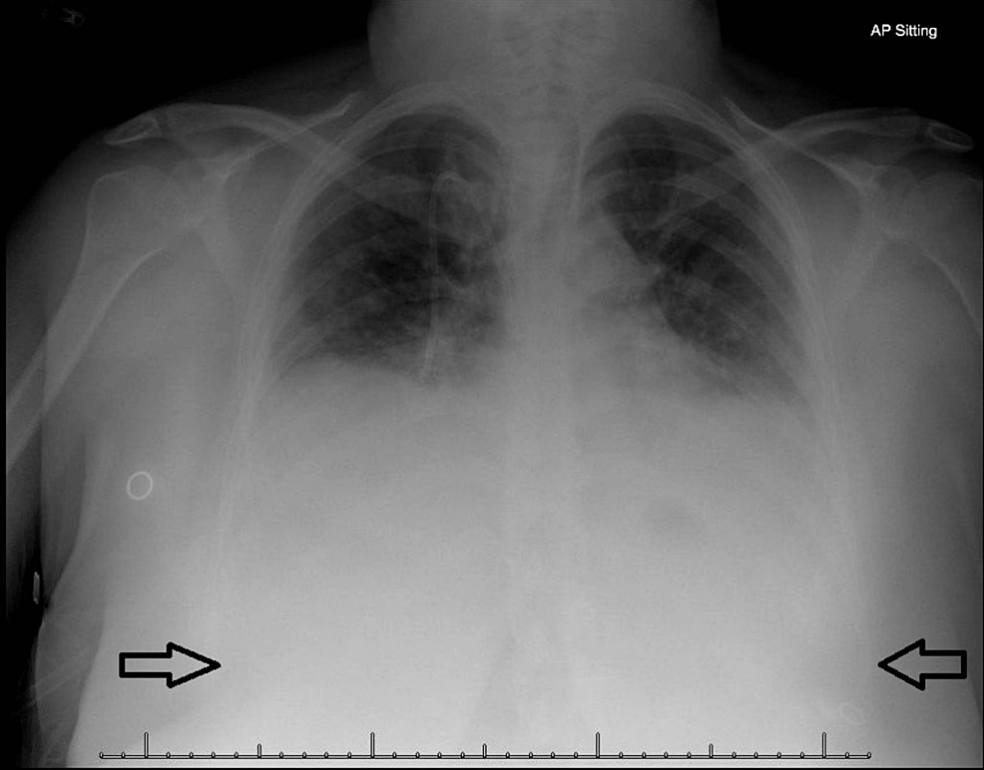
Chest radiograph with the arrows depicting moderate bilateral pleural effusions.
